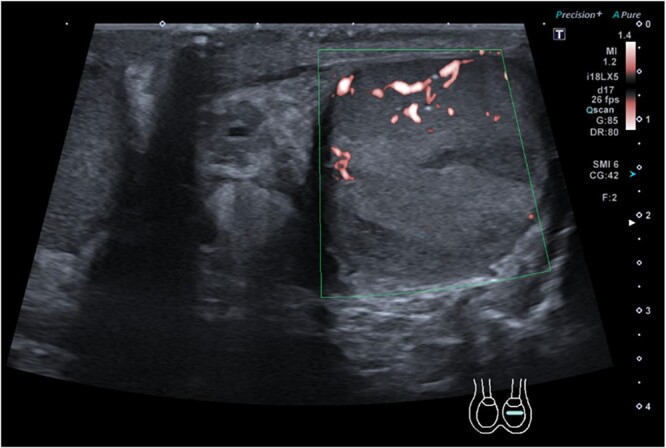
Ultrasound sonography of left testicle demonstrating wedge segmental infarcts which are avascular on colour doppler.
